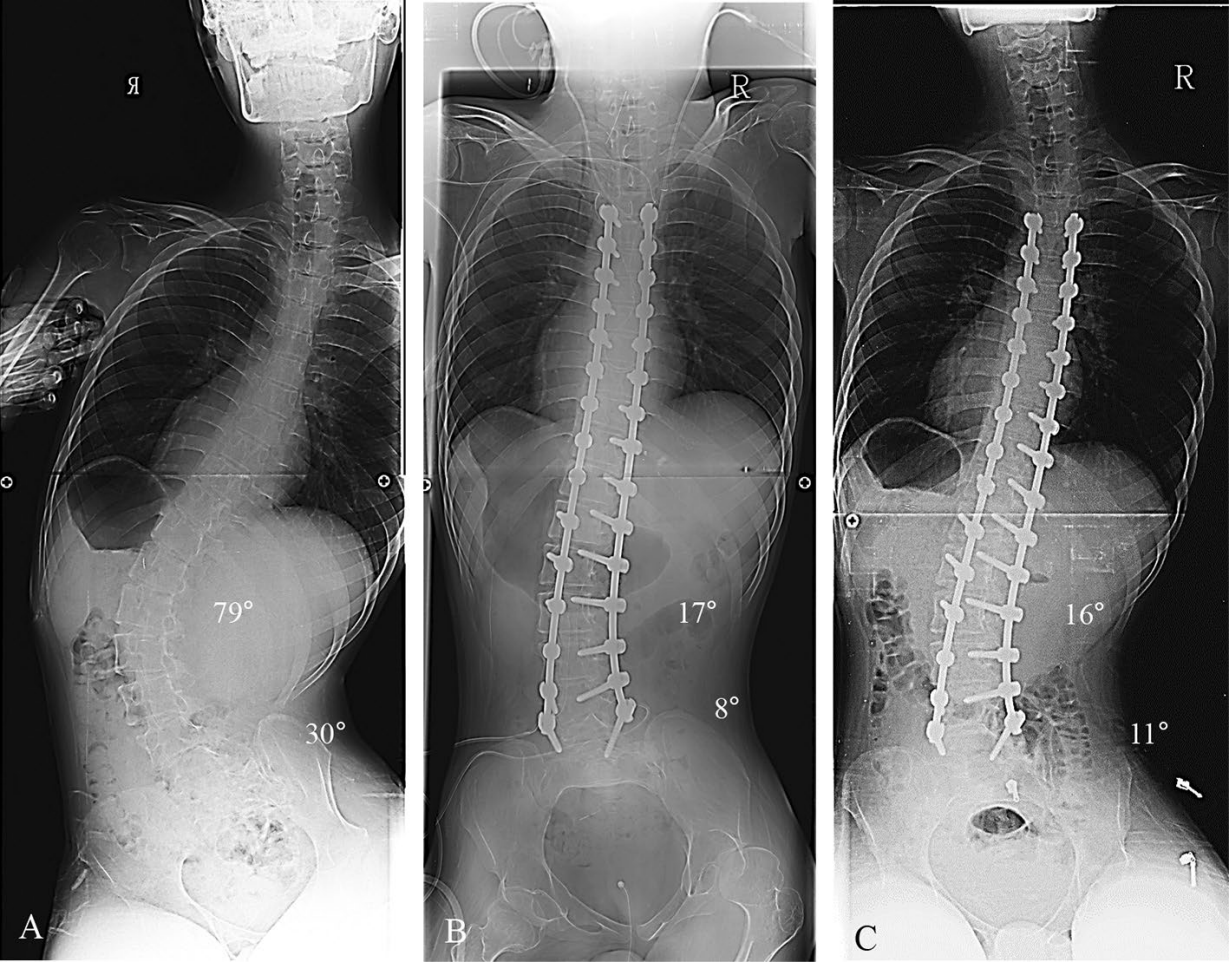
Deformity correction from T4 to L5 in patients with neuromuscular scoliosis (NMS). (A) A 16-year-old male patient who diagnosed with Duchenne muscular dystrophy showed neuromuscular scoliosis. The whole-spine anterior–posterior image showed 79° of scoliosis deformity, and pelvic obliquity was 30° as measured by pelvic tilting angle. (B) The immediate postoperative radiograph showed 17° of the Cobb’s angle (78.4% of correction rate) and 8° of the pelvic tilting angle (73.3% of correction rate, which are not performed by spinopelvic fixation). (C) The last follow-up Cobb angle showed 16° and pelvic obliquity was 11°.
